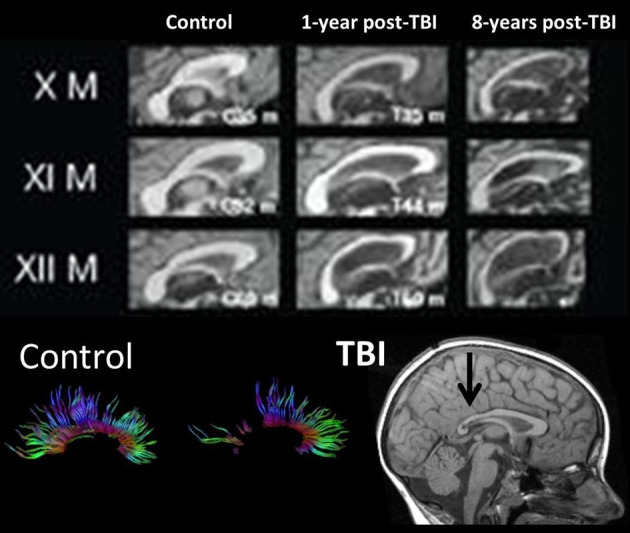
(TOP) Mid-sagittal section through the corpus callosum showing initial atrophy 1-year from TBI, but increasing atrophy within this WM structure expressed over the next 8 years, indicating late neurodegenerative effects on WM. Images reproduced with permission from Tomaiuolo et al. (2012) and Elsevier Science. (BOTTOM) Corpus callosum tractography extracted from DTI in a control, compared to a child with severe TBI. The mid-sagittal MRI shows gross thinning of the posterior corpus callosum (dark arrow) but DTI actually demonstrates that this reduced area actually has regions of no DTI-identifiable aggregate WM tracts. Adapted from Wilde et al. (2006b) used with permission from Mary Ann Liebert Publishing.
